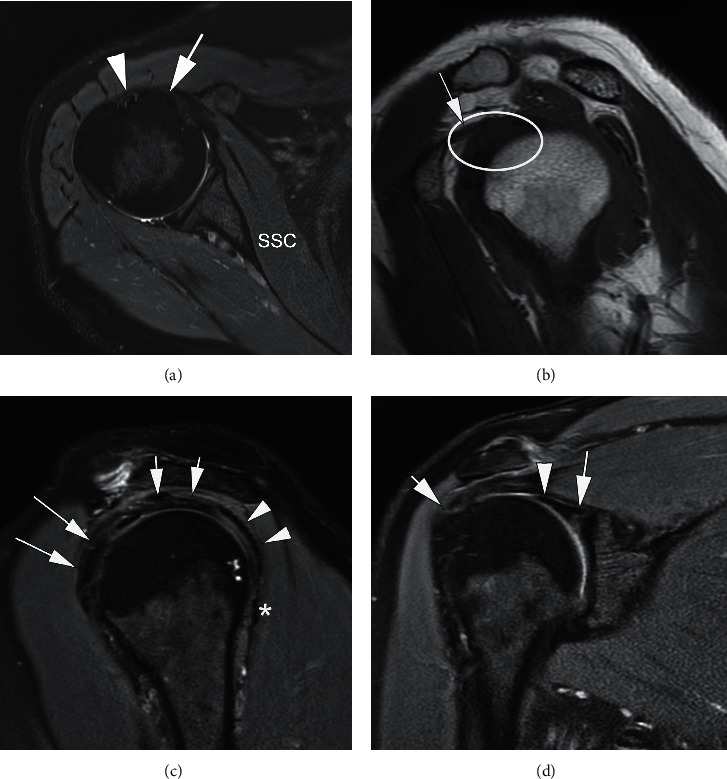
Normal MRI of the right shoulder. Axial T2-weighted fat-suppressed (FS) MRI (a) demonstrates the subscapularis muscle (SSC) traversing along the anterior aspect of the scapula to insert on the lesser tuberosity of the humerus (arrow). The long head of the biceps tendon (arrowhead) is correctly positioned in the bicipital groove at this level. Sagittal oblique T1-weighed MRI (b) provides a view of the rotator interval (ellipse) with visualization of the coracohumeral ligament (arrow). Sagittal oblique T2-weighed FS MRI (c) shows the lateral subscapularis (long arrows), supraspinatus (short arrows), infraspinatus (arrowheads), and teres minor (star) tendons close to their humeral attachment. Coronal T2-weighted FS MRI (d) shows the biceps anchor (arrow head) and the proximal horizontal portion of the long head of the biceps tendon close to its attachment at the glenoid (long arrow). *Note.* Tendinosis of the supraspinatus attachment is reflected as intratendinous hyperintensity (short arrow).
